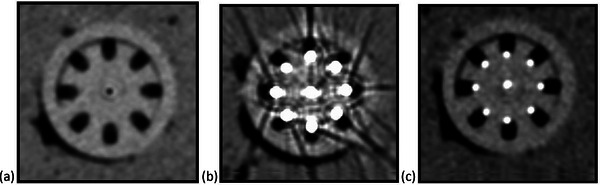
Use of radio opaque markers to identify needle location. Various methods of identifying the needle location, and therefore the source position within the needles, for applicator digitization on CT scans are shown here. Panel (a) shows the CT scan with no markers in the needles, (b) shows the CT artifacts present when using the VMS‐numbered markers, and (c) shows a CT scan using a fish wire marker in each channel. VMS, Varian Medical Systems.
